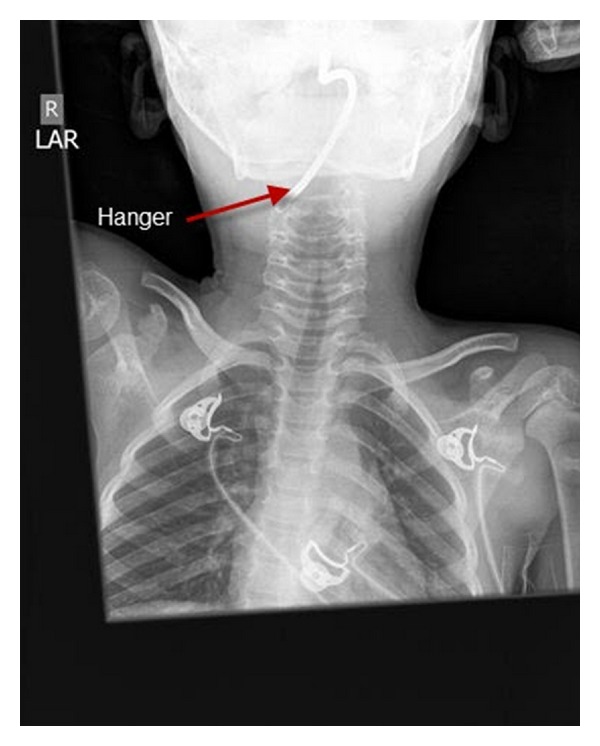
Anterior/Posterior neck X-ray again showing foreign body.
